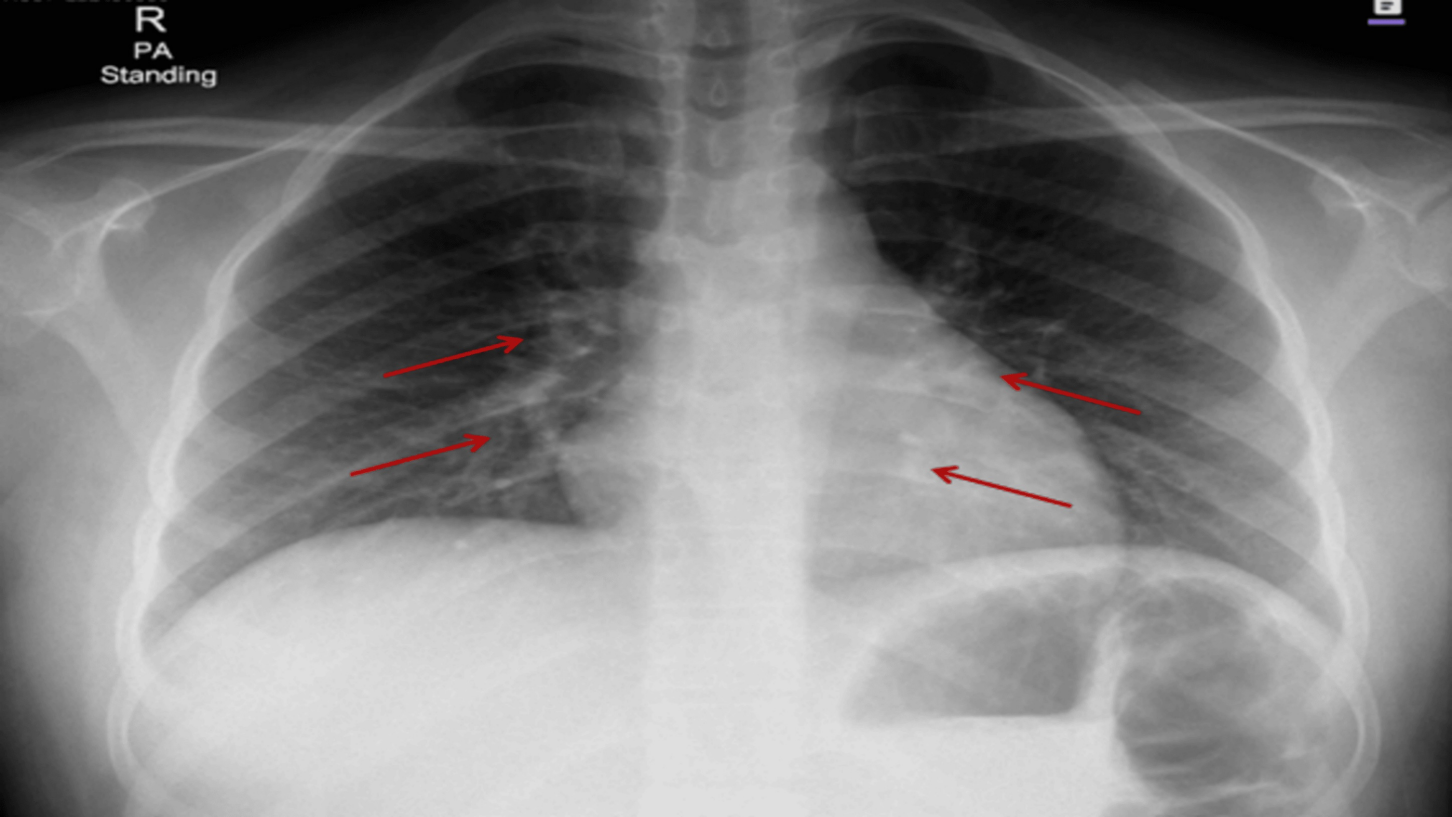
Posteroanterior chest radiograph showing prominent bilateral perihilar bronchovascular markings (arrowed) with a normal cardiac silhouette and no radiographic evidence of pleural effusion.
